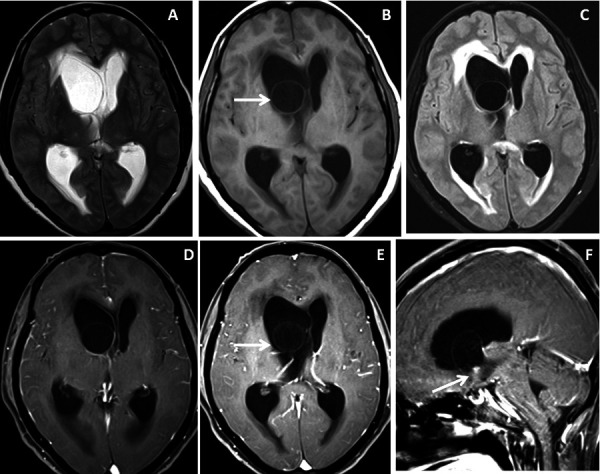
Brain magnetic resonance imaging of 50 years female with acute severe headache with acute neurological status and semi-comatose state. (A-C) Axial T2WI, FLAIR and T1WI images show a thin-walled T2 hyperintense cystic lesion in the frontal horn of the right lateral ventricle occluding the right-sided foramen of Monroe(arrow) with marked supratentorial hydrocephalus with periventricular CSF seepage. (D-F) Axial and sagittal post-gadolinium T1W images show smooth peripheral cyst wall enhancement (arrow) with a small enhancing mural nodule inferiorly (arrow in image F).
